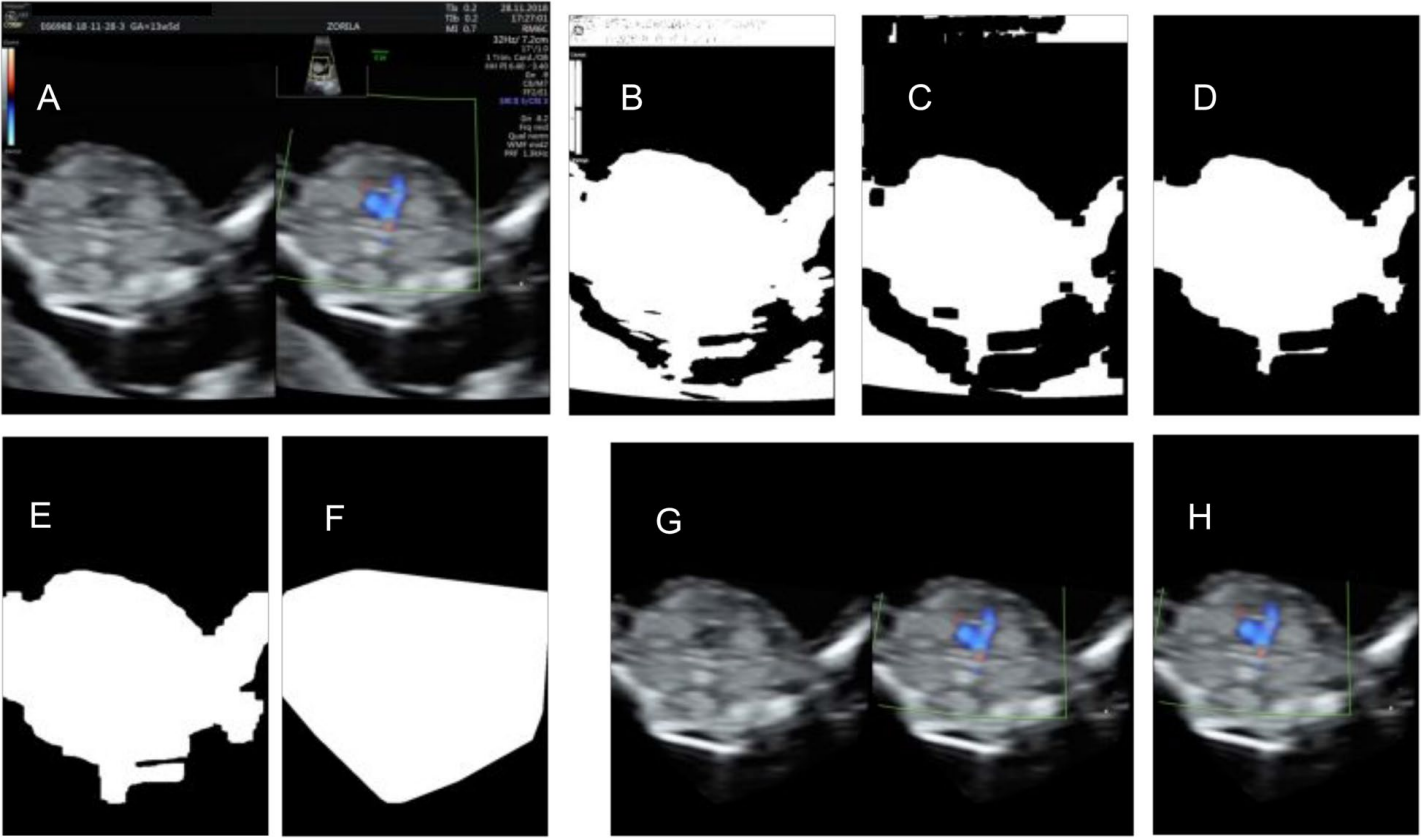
Examples in which background is removed from the initial image (A), intermediary images are obtained for one side (B-F), and either both views are kept (G), or the Doppler color side is used alone (H)
